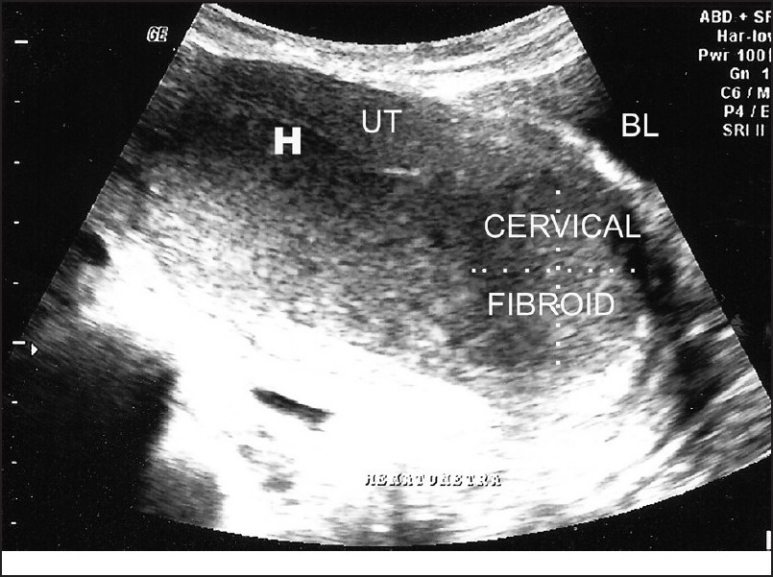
Transabdominal ultrasound revealed an enlarged uterus and cervical fibroid with iso-to hyperechoic collection within the endometrial cavity; BL-bladder, UT-uterus, H-hematometra
